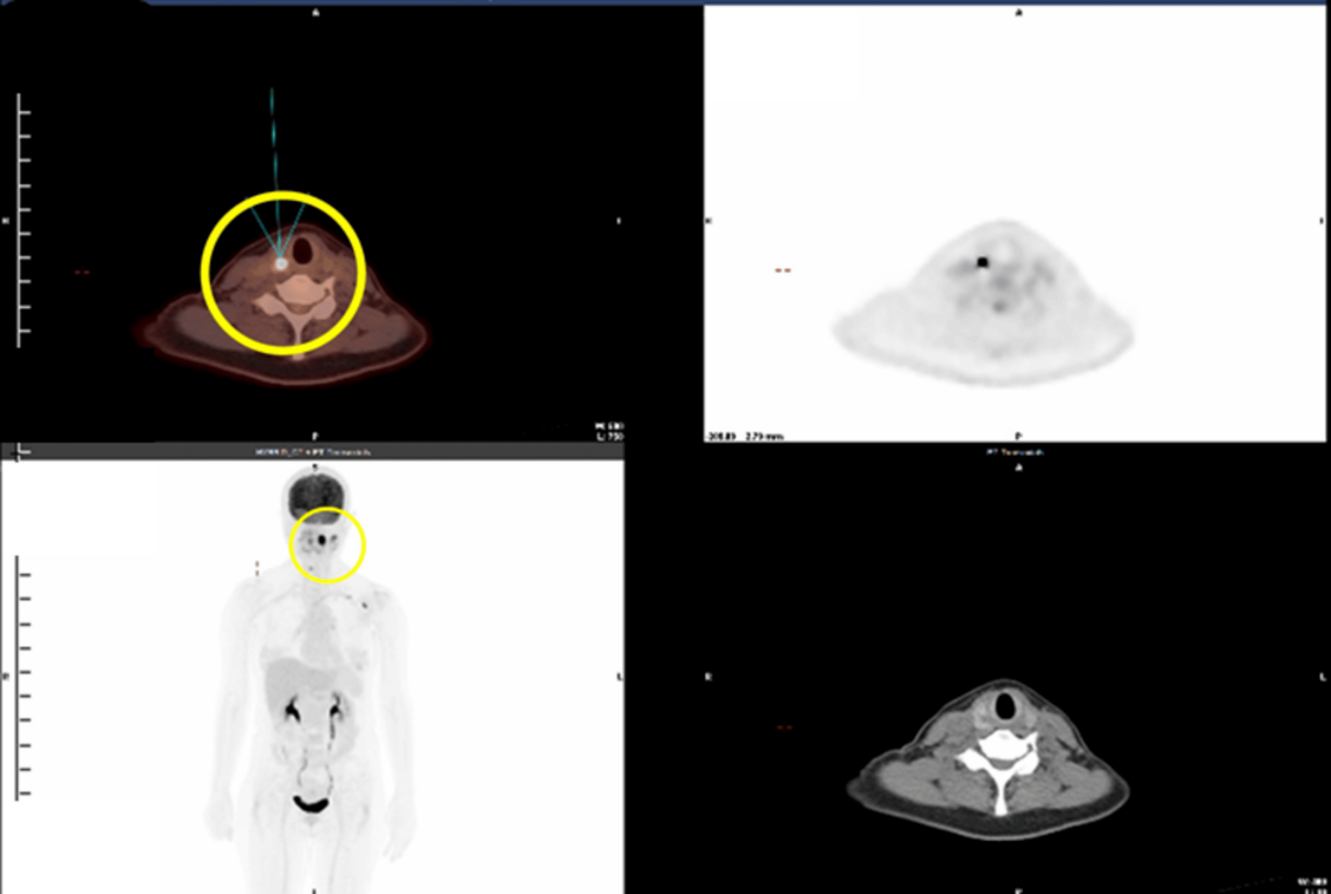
PET/CT showing an abnormal hypermetabolic nodule (about 1 cm, SUV max: 50.89) in the right thyroid lobe PET/CT: positron emission tomography/computed tomography; SUV max: maximum standardized uptake value
